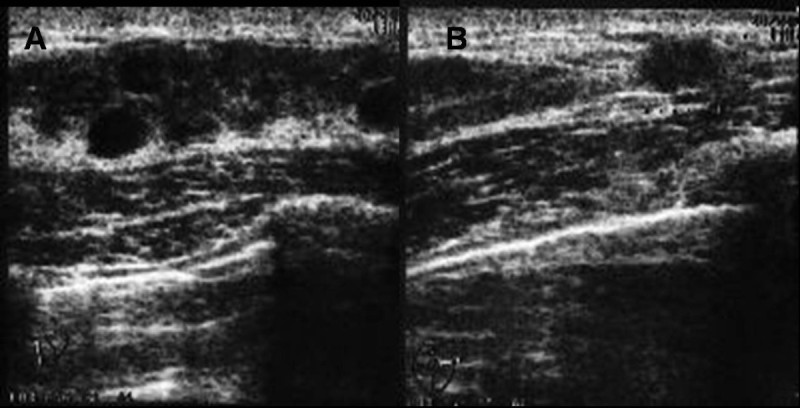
A Ultrasonography of induration of the left breast demonstrates a low-echoic area measuring 5 × 5 × 1 cm with multiple cysts.B Ultrasonography of the hard lump demonstrates an irregular hypoechoic mass measuring 0.6 × 0.6 × 0.4 cm near the low-echoic area described above.
